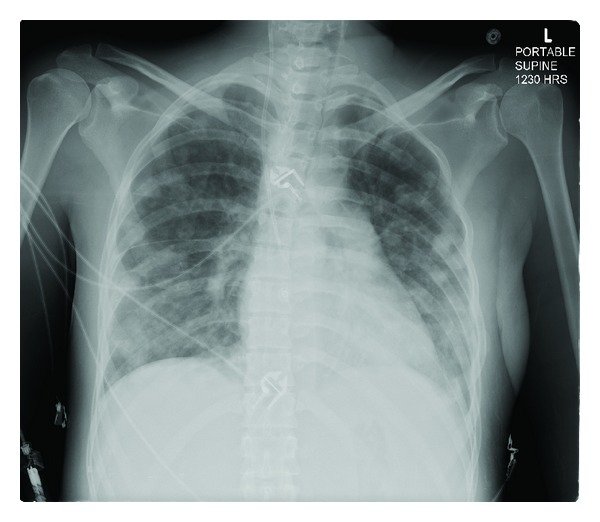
Chest X-ray showing pulmonary septic emboli.
